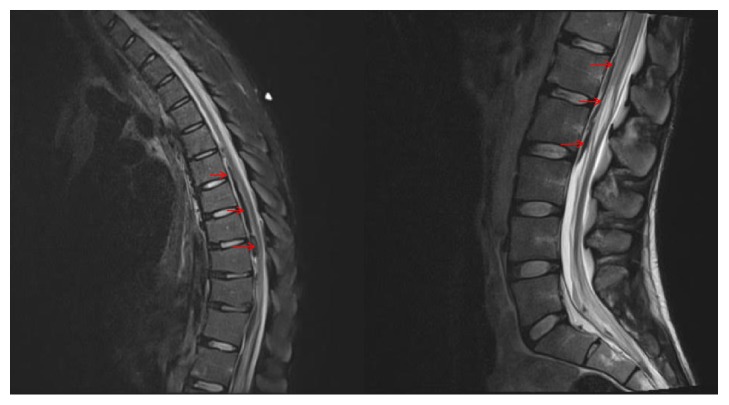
MRI showing thoracic (*left*) and lumbar (*right*) subdural hematomas (arrows).
